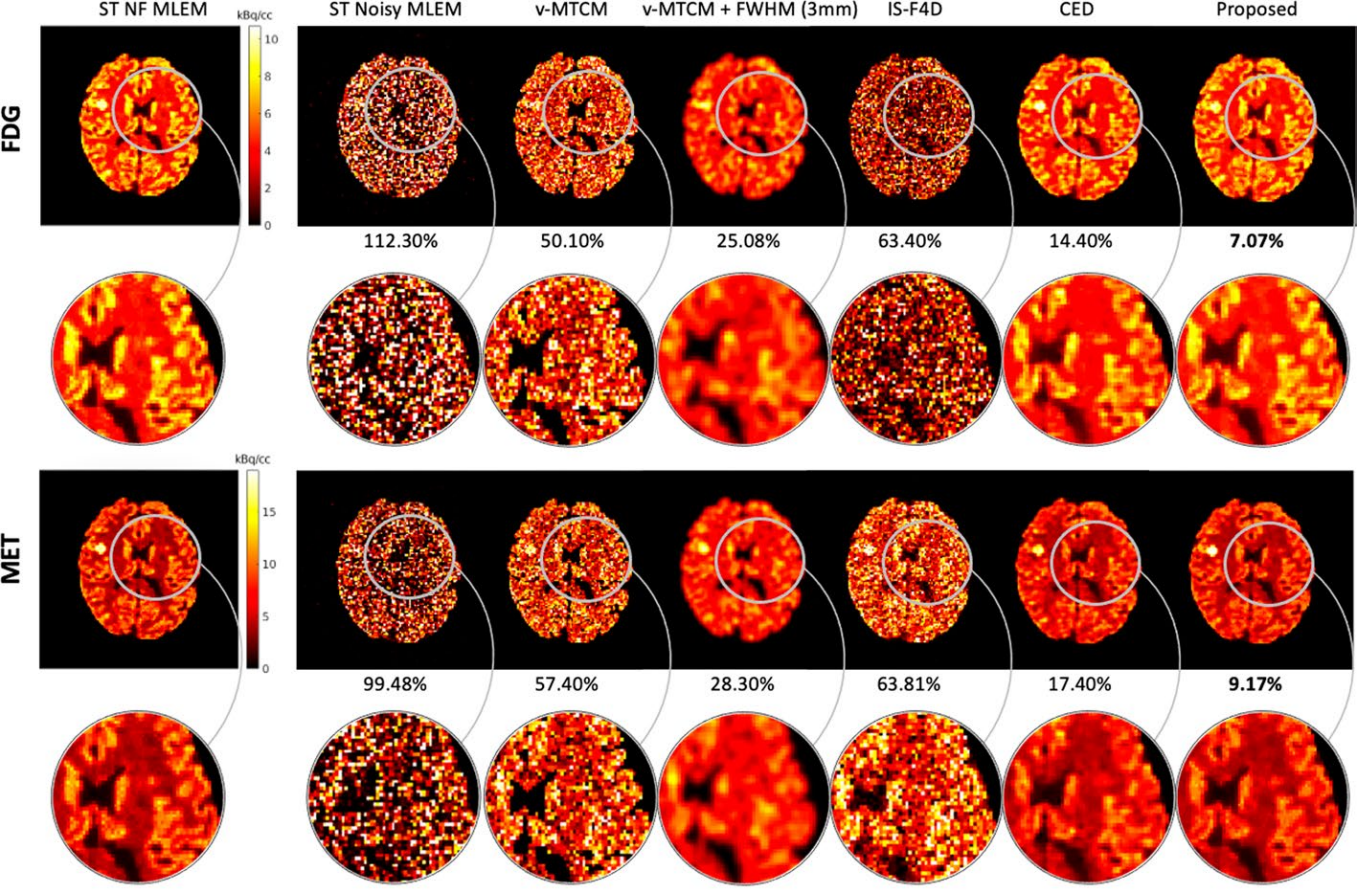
Frame 14 ($$t = 6.25$$ min) of the separated tracer activity images for FDG and MET in a test example using v-MTCM, v-MTCM+FWHM (3 mm), IS-F4D, the CED [37], and the proposed kinetic model-informed unrolled deep network. NRMSE values of each separated image are shown at the bottom. For plotting only, we limit the colour scale of the figures to fall within the same range as the reference images shown in the first column
